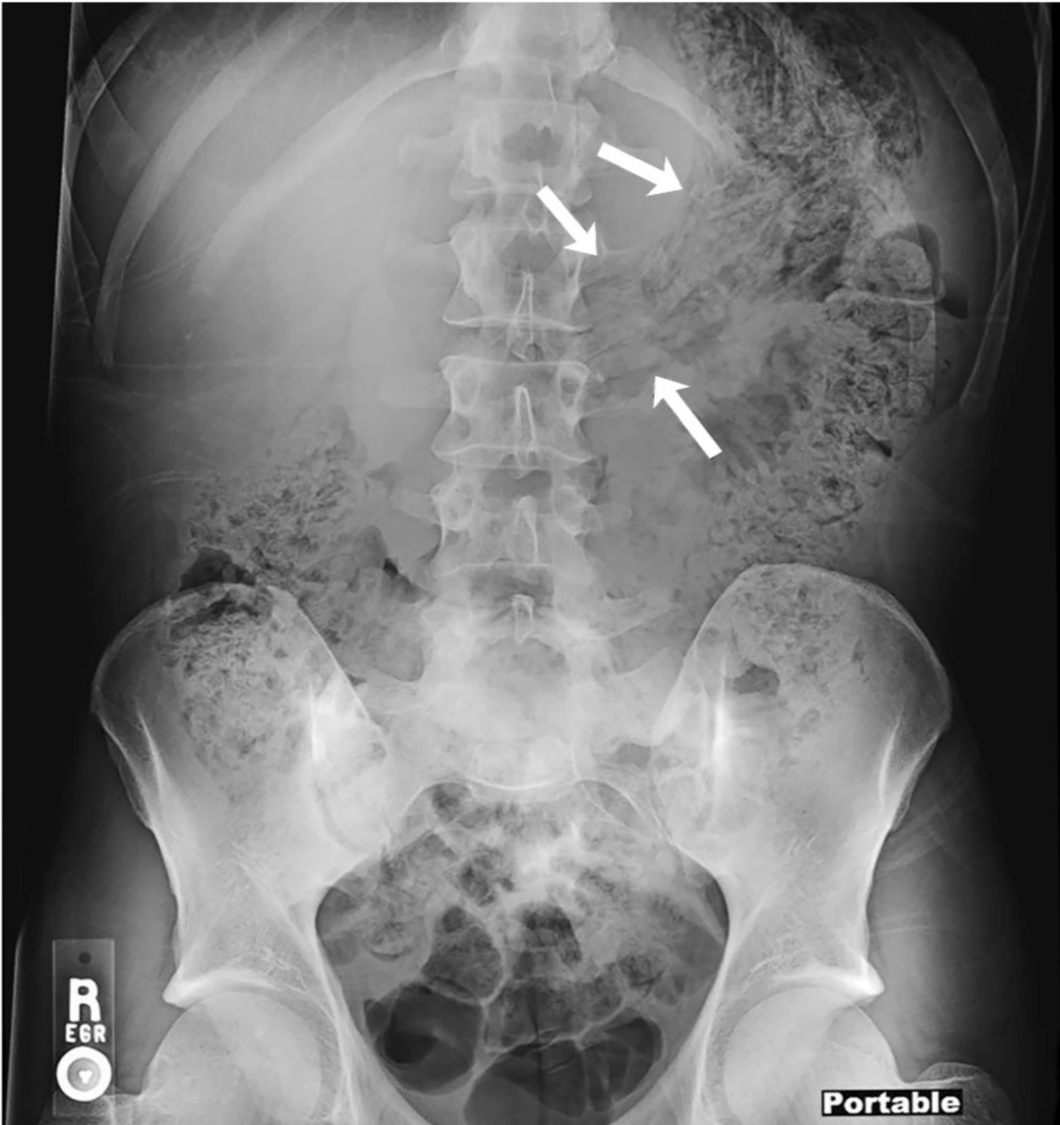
Abdominal X-ray findings demonstrating amorphous material with a linear appearance in the stomach (marked with white arrows).
